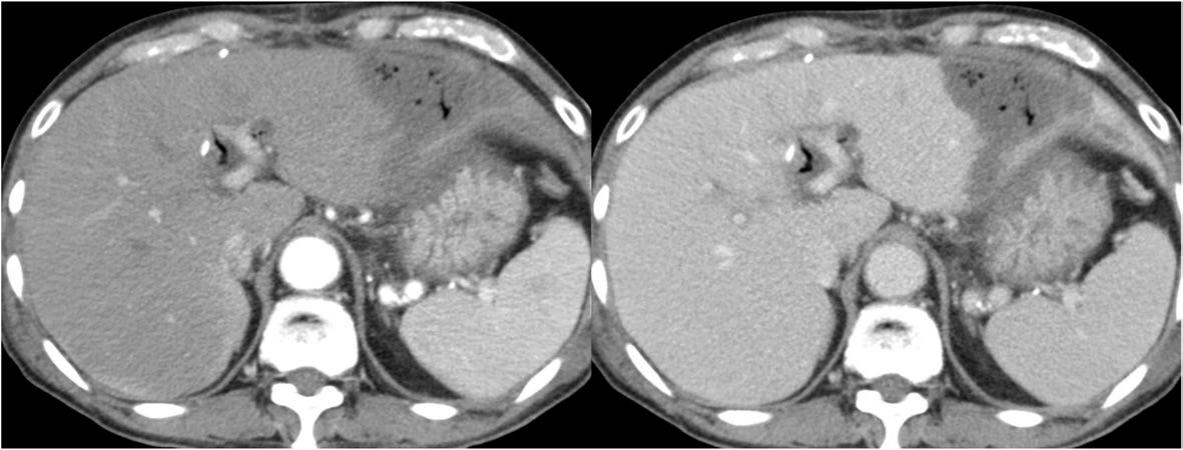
Hepatic infarction at segments 2 and 3 was observed in case 6 on the arterial and delayed phases of CTA after endovascular therapy
